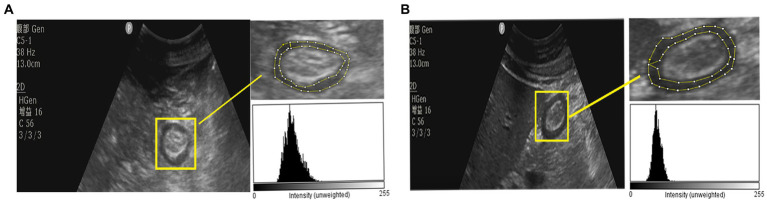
The POCUS image of gastric antrum echodensity between effective group and ineffective group. (A) The gastric antrum ultrasound image of a patient which was in ineffective group. (B) The gastric antrum ultrasound image of a patient which was in effective group.
